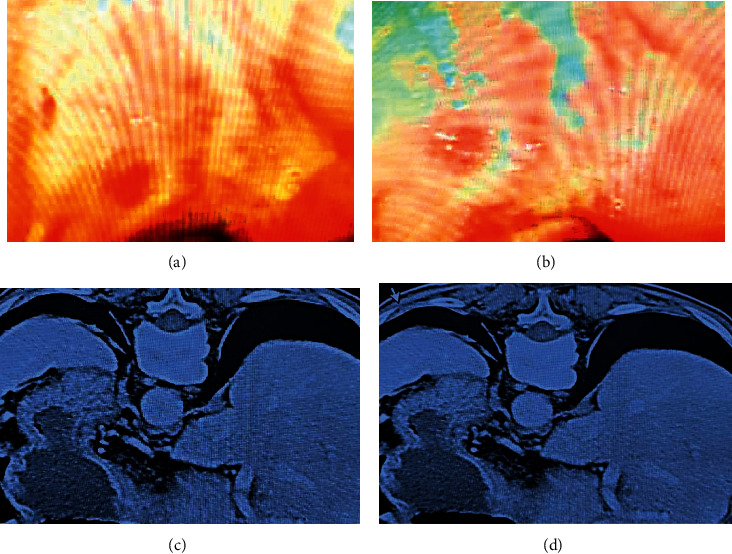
Imaging data of a 70-year-old woman with persistent pain and discomfort in the upper abdomen for 3 weeks before visiting the hospital, and with the worsened pain in the past 1 week. (a) and (b) were the results of gastroscopy, while (c) and (d) were CT images.
